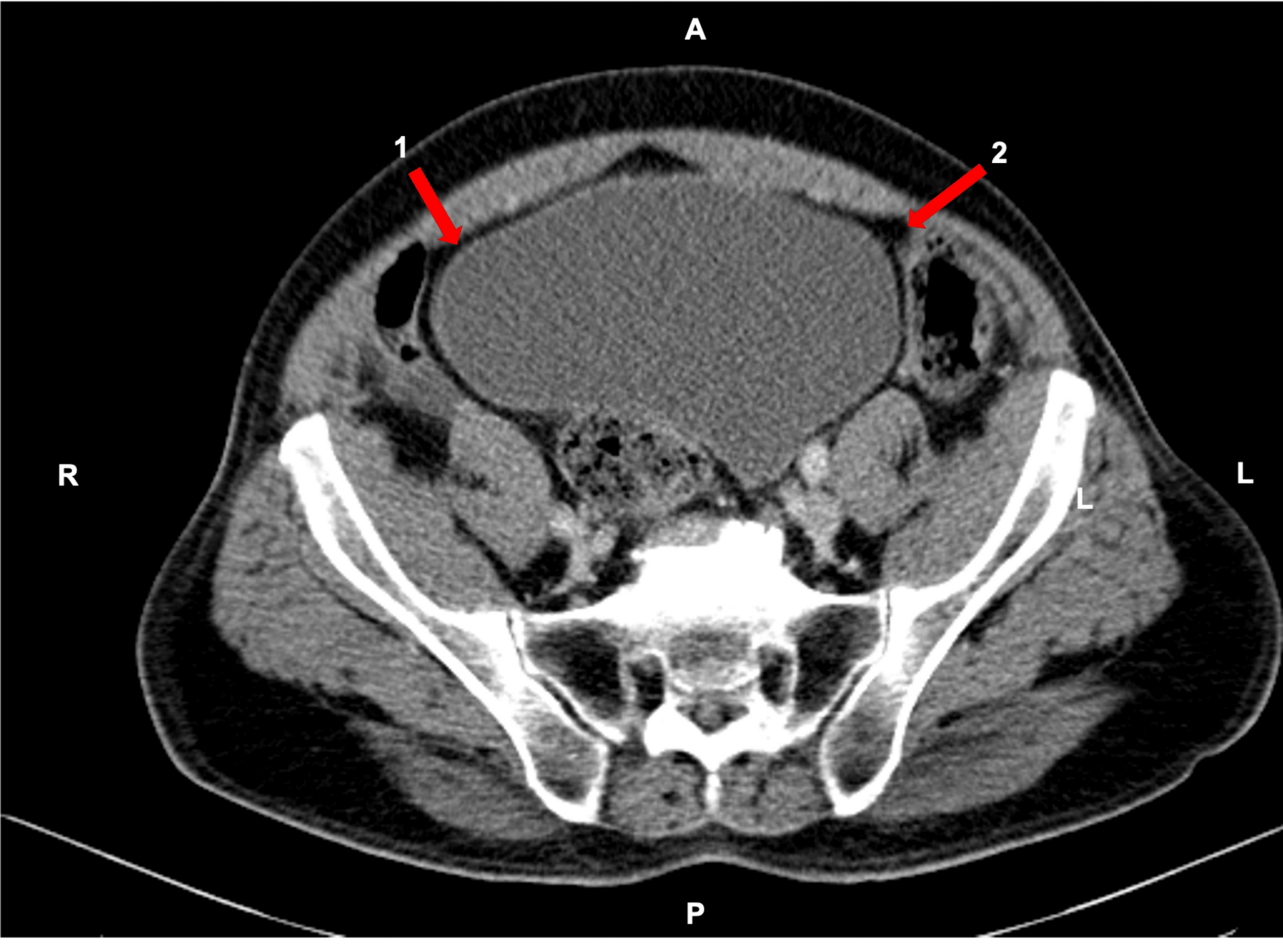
Axial section on contrast-enhanced CT of the abdomen and pelvis Bladder wall intramural gas favoring the diagnosis of EC. (1) and (2): Gas bubbles in the bladder wall. (A) Anterior. (B) Posterior. EC, emphysematous cystitis; CT, computed tomography
